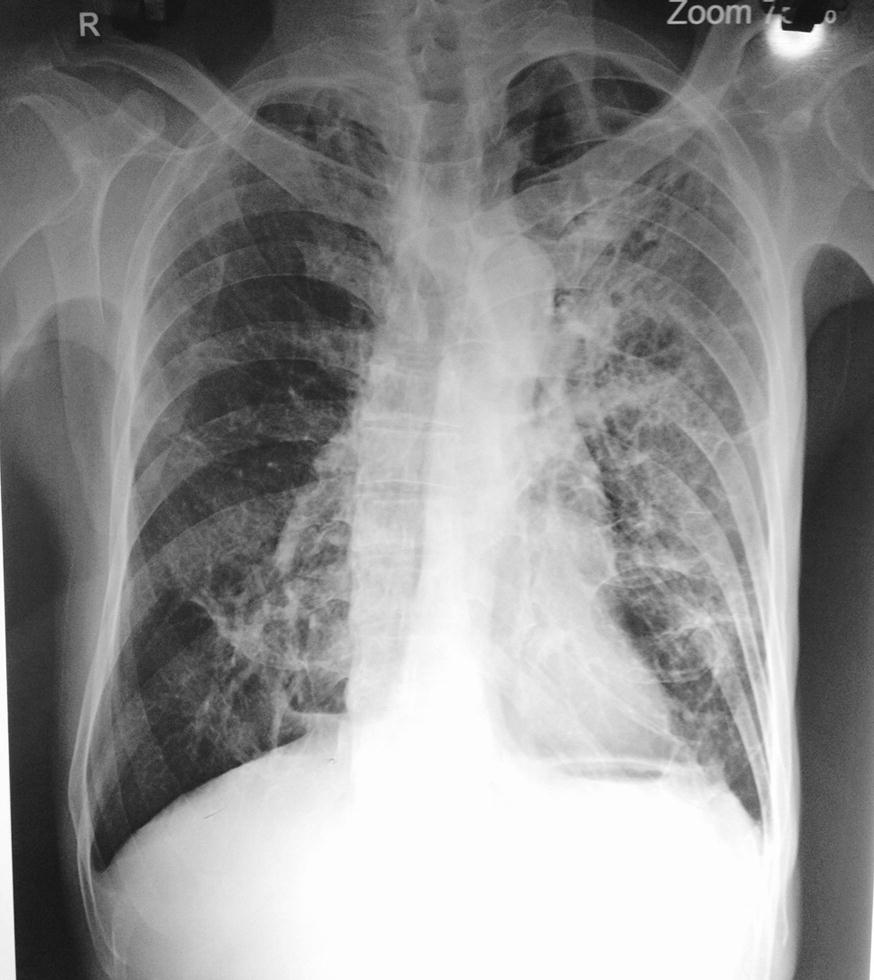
posterior–anterior (PA) view chest x-ray: left perihilar opacity with fibrotic changes
